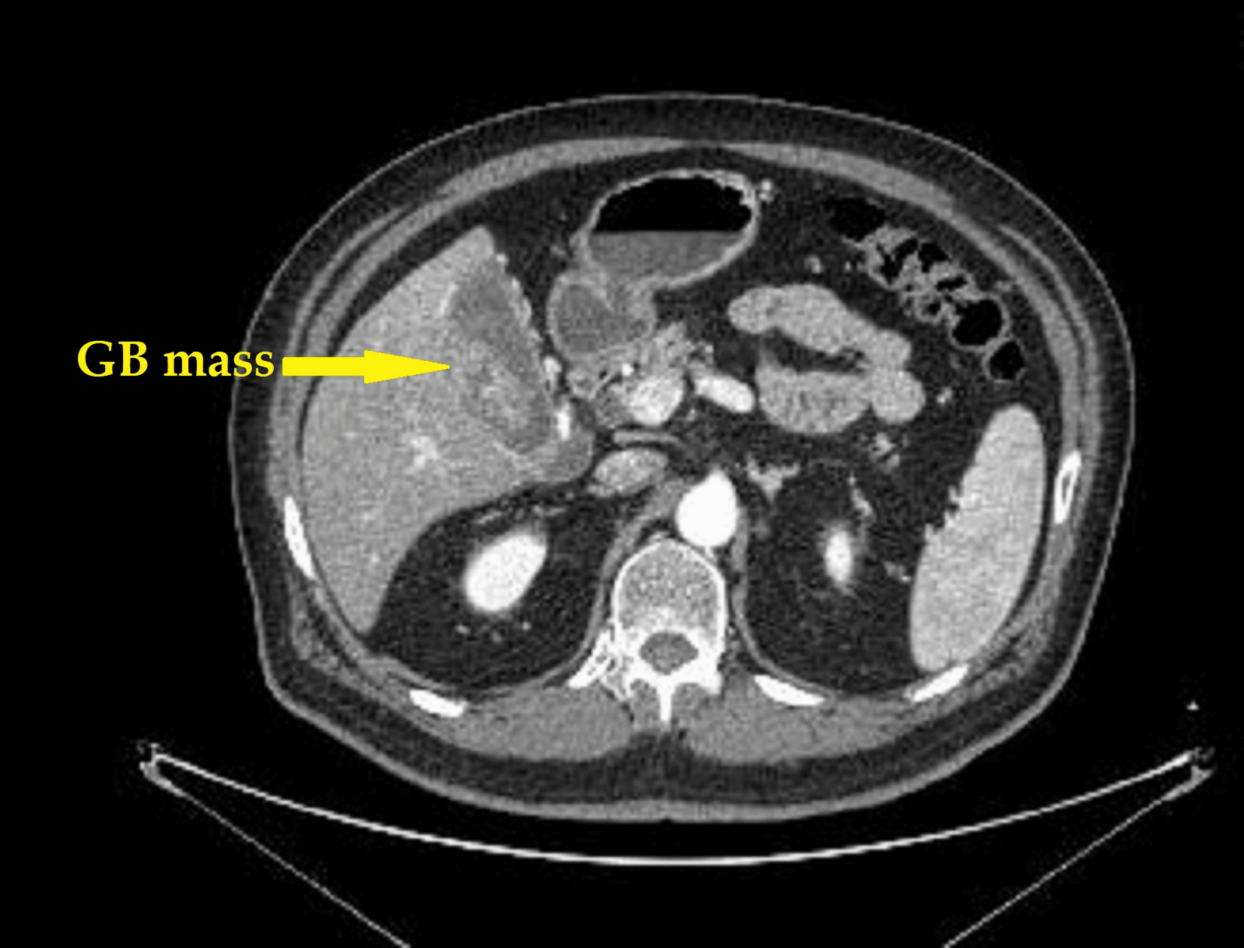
A CT scan showing a gallbladder mass (arrow) and an enlarged gallbladder (5*12.5 cm) in size showing multiple ill-defined, intensely enhancing, irregular masses; most likely gallbladder cancer.
